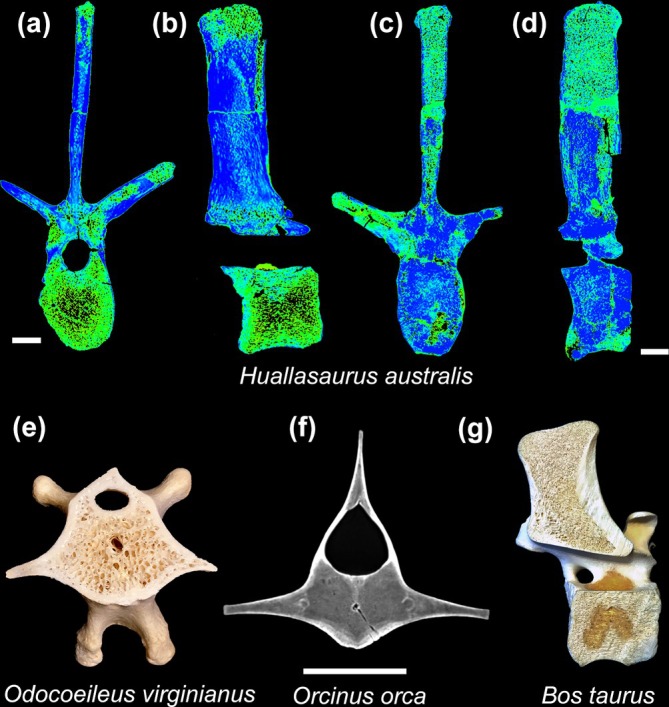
Comparison between the dense trabecular tissue in the vertebrae of the hadrosaur *Huallasaurus australis* (a–d) and the internal structures in modern mammals (e–g). (e) Bone cross section of *Odocoeileus virginianus*, a North American deer (unnumbered; courtesy of Mathew Wedel). (f) CT scan of *Orcinus orca*, the orca whale (lumbar; CEM 038977; courtesy of Gabriel L. Gomes). (g) Bone cross section of *Bos taurus*, the domestic bull (unnumbered; courtesy of Mathew Wedel). Transverse view in (a, c, e, f). Parasagittal view in (b,d,g). Scale bar in (a–d) = 20 mm; in (f) = 10 mm. (e,g) Not to scale.
